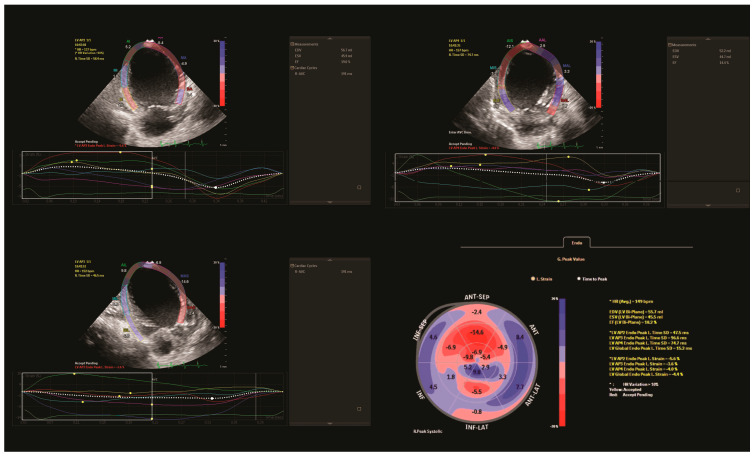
Preoperative speckle-tracking analysis. LV longitudinal strain from the apical two, three and four-chamber view. Time-strain curves show a pathological deformation in the anterior, anterolateral, and inferior LV regions with a hypokinetic septum. Final bull’s-eye plot reveals low global longitudinal strain with severe impairment of both left and right coronary artery territories that supply the left ventricle. AP2: apical two-chamber view; AP3: apical three-chamber view; AP4: apical four-chamber view; EDV (bi-plane): end-diastolic volume (bi-plane); ESV (bi-plane): end-systolic volume (bi-plane); EF (bi-plane): ejection fraction (bi-plane); Global Endo Peak L. Strain (GLS): global longitudinal strain; and HR: heart rate.
